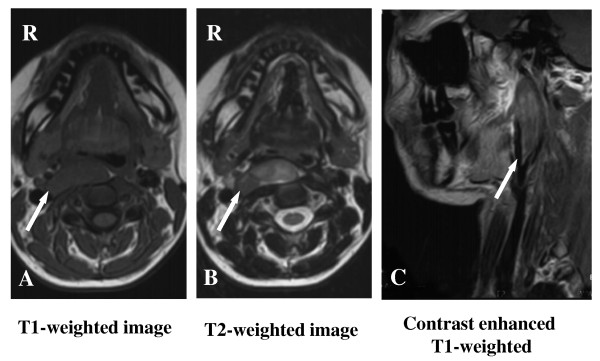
MRI performed before surgical resection. (A) MRI showing that the tumor has low-signal intensity on T1-weighted images. (B) Heterogeneous high-signal intensity on T2-weighted images. (C) The tumor exhibits an uneven contrast effect on T1-weighted coronal images. Arrows indicate a tumor.
